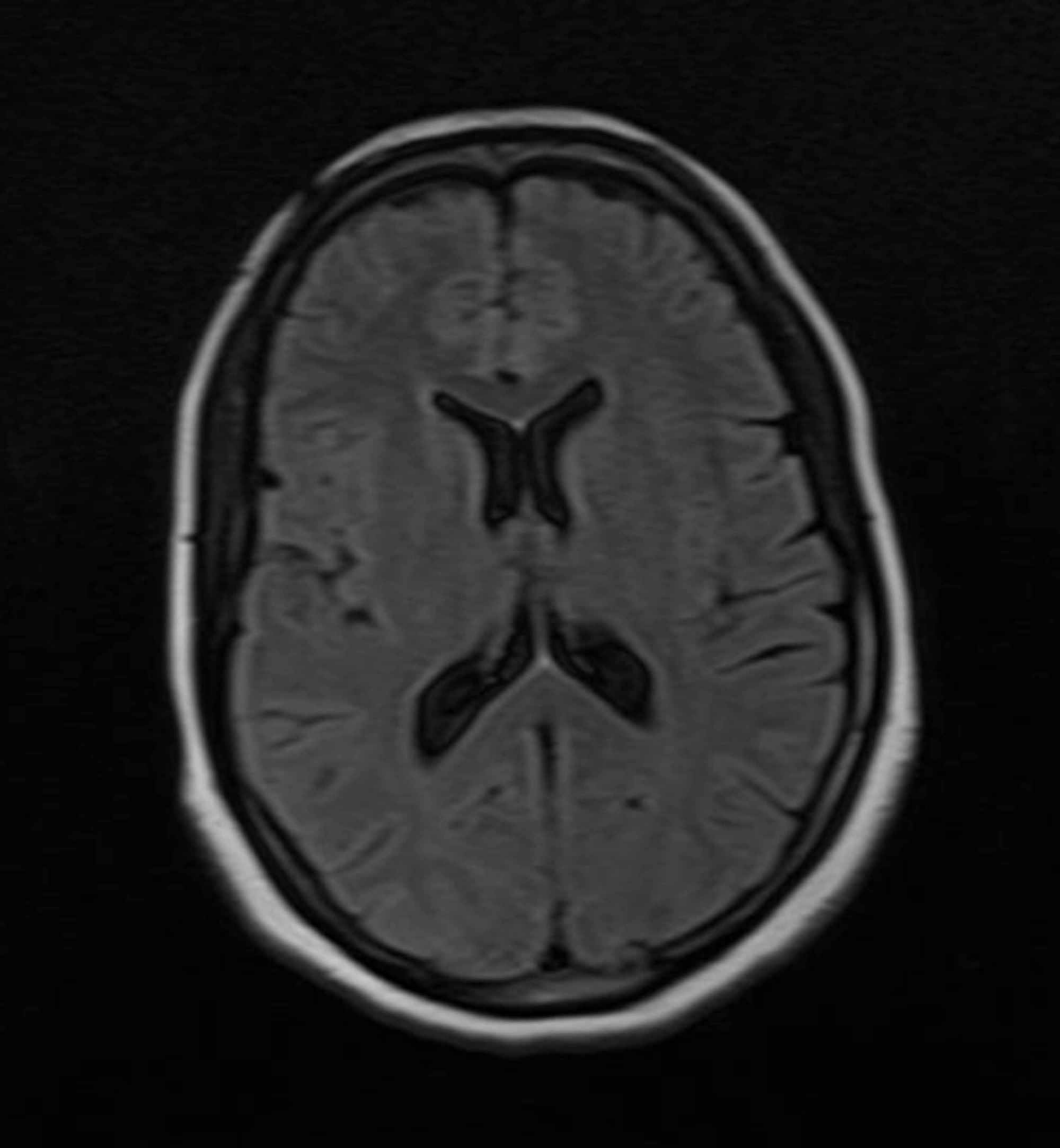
Non-contrast MRI brain (axial view) MRI = Magnetic Resonance Imaging
